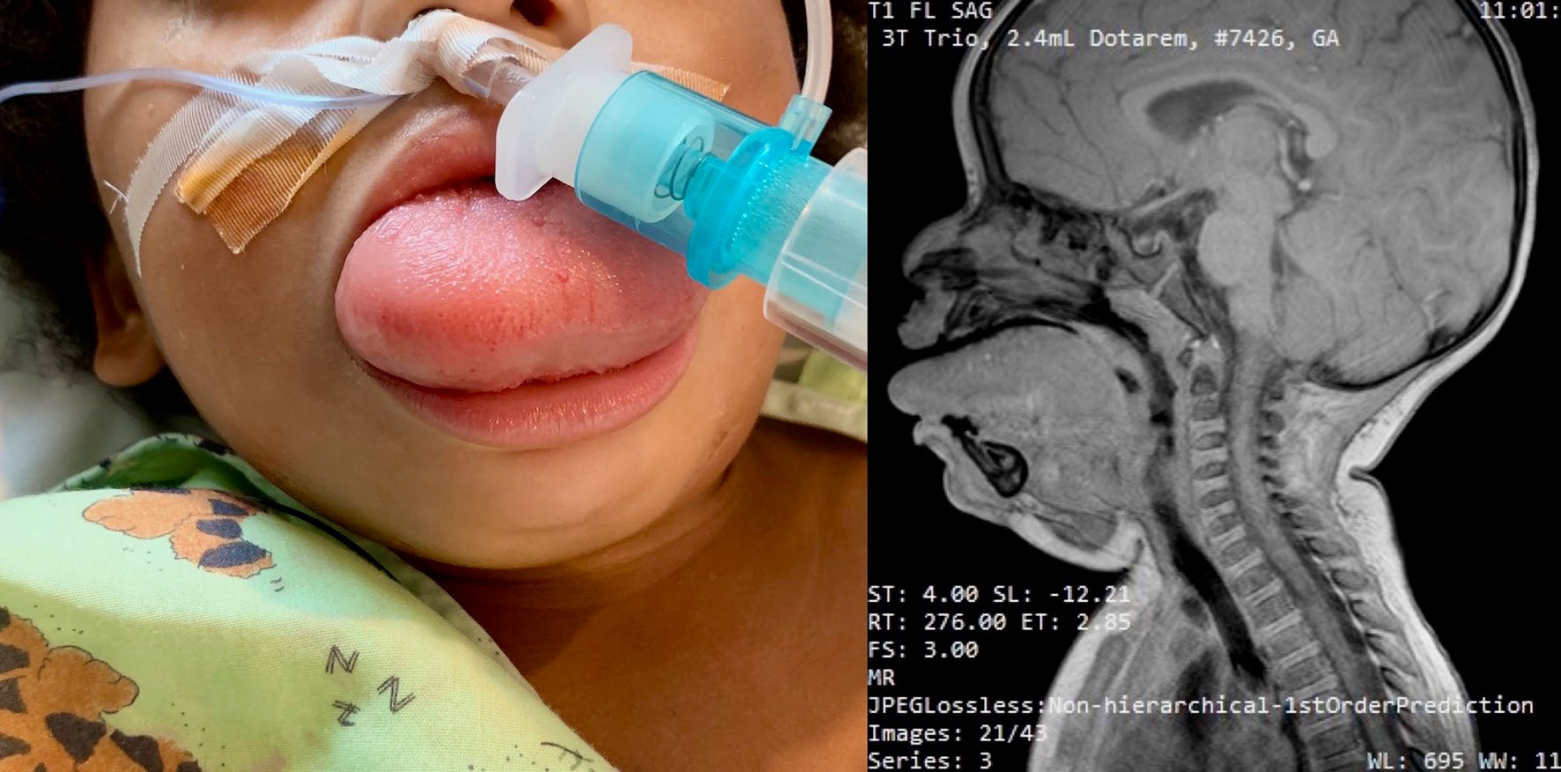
Child with lymphatic tongue malformation demonstrating severe macroglossia, and magnetic resonance imaging demonstrating soft tissue lymphatic infiltration
